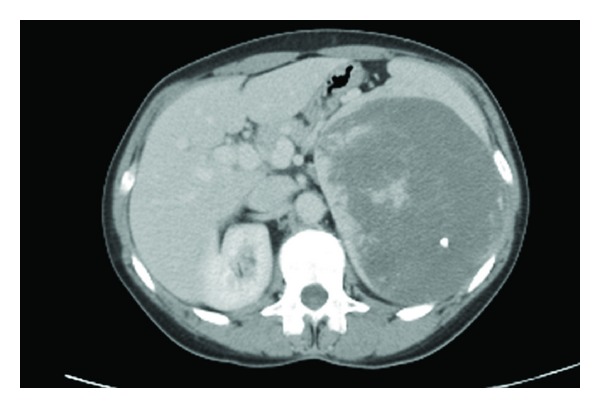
CT image of large left upper quadrant mass with evidence of necrosis and a calcific focus.
